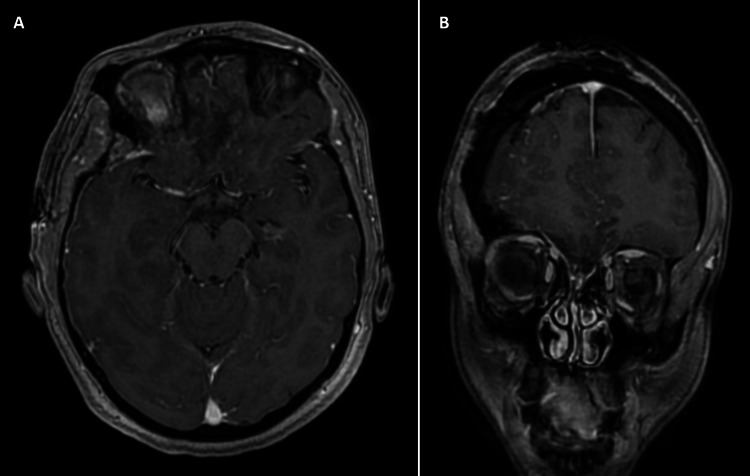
Postoperative MRI Postoperative gadolinium-enhanced T1-weighted images in axial (A) and coronal (B) views showing complete resection of the mass lesion.
